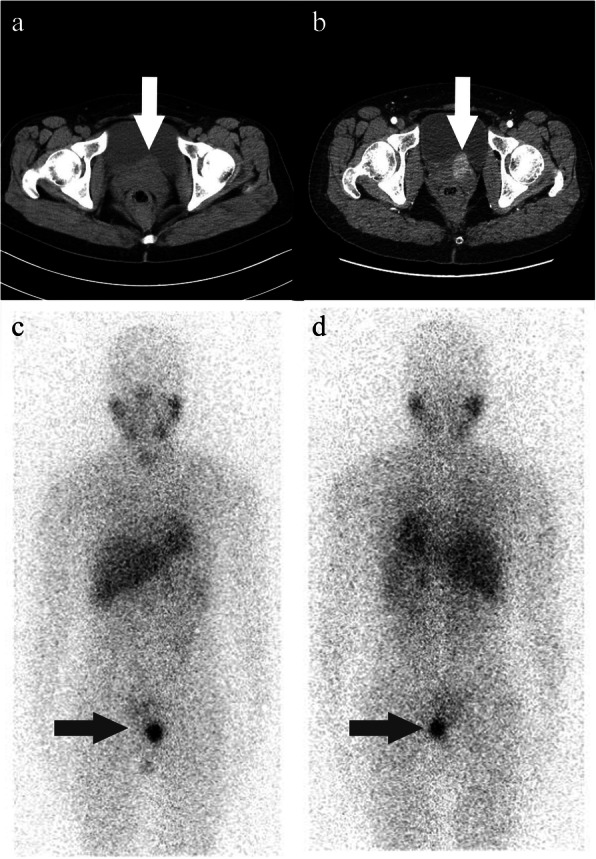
CT and ^131^I-MIBG findings of vaginal paraganglioma. a: abdominal and pelvic CT scan showed a mass (white arrow) on the left side of the vagina, protruding into the bladder; b: enhanced scan showed obvious enhancement of the tumor (white arrow); c and d: ^131^I-MIBG scan (anteroposterior and posteroanterior position respectively) showed abnormal increase of radioactive uptake on the left side of perineum area (black arrow)
